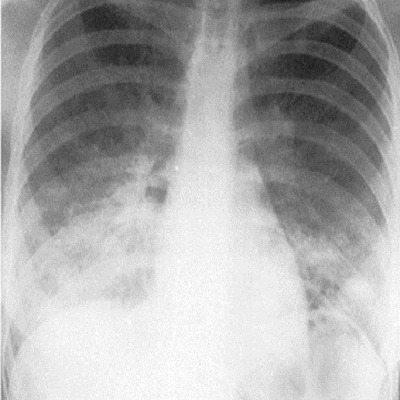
Radiographie du thorax évoquant une infiltration des bases pulmonaires
